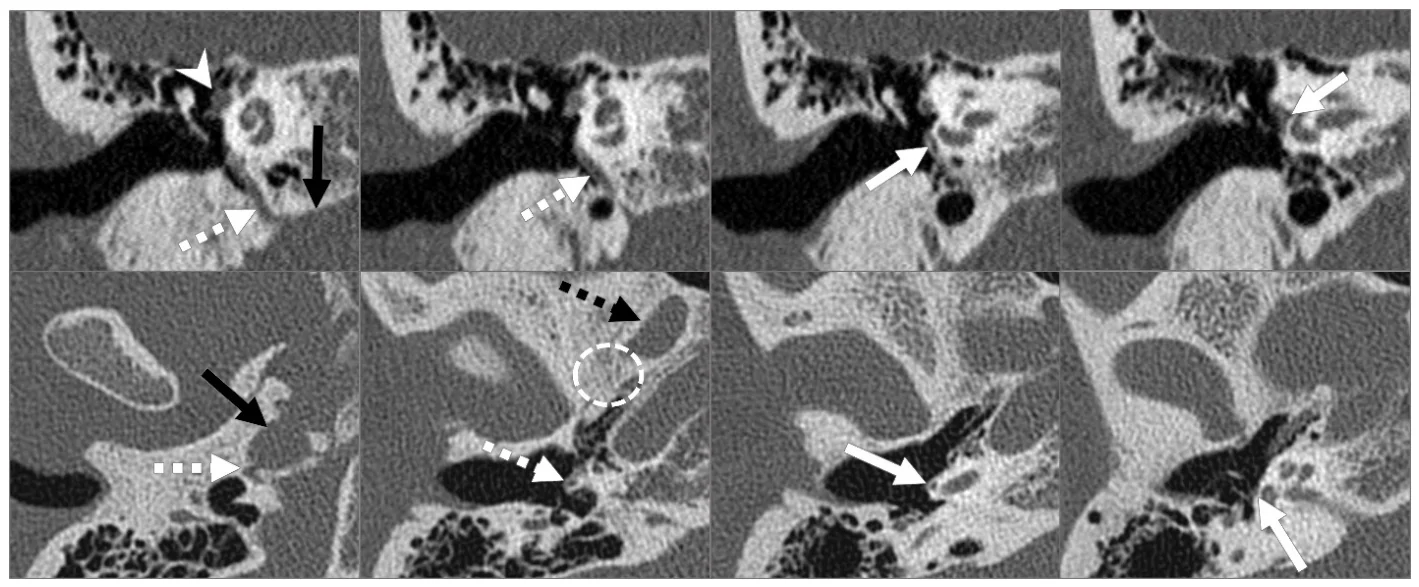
Persistent stapedial artery. Coronal (upper row) and axial (lower row) HRCT images of the temporal bone demonstrate the absence of the foramen spinosum (white circle) posterior to the foramen ovale (dashed black arrow), indicating that the middle meningeal artery arises from the ICA instead of the ECA. A small vascular canal (dashed white arrows) arising from the petrous ICA (black arrows), corresponding to the caroticotympanic artery canal, extends superiorly along the cochlear promontory and the stapes footplate, consistent with a persistent stapedial artery (white arrows). There is enlargement of the anterior aspect of the fallopian canal, suggesting a persistent stapedial artery distally formed by the superficial petrosal artery (white arrowhead).
